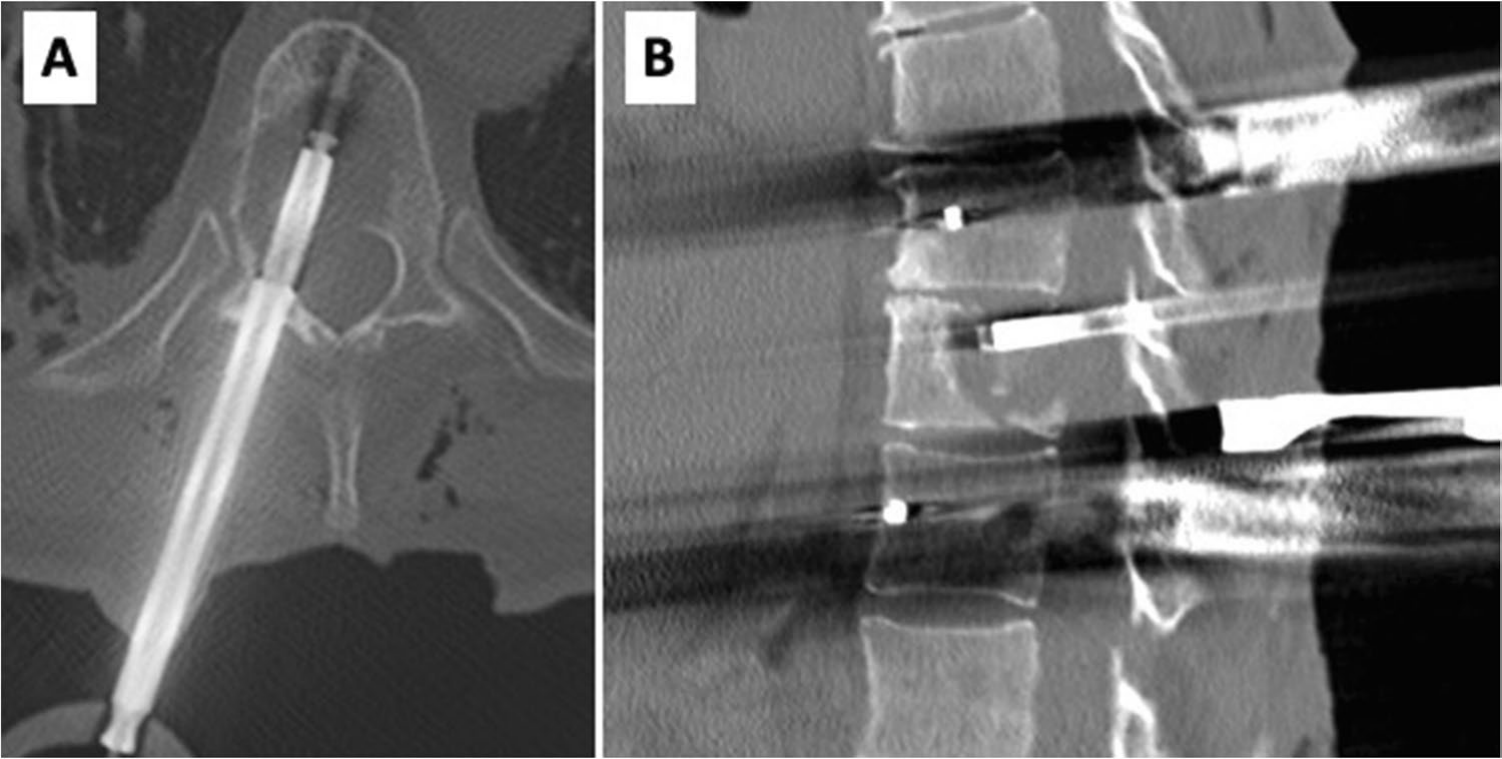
A) axial CT scan with intravertebral placement of guide and needle applicator in axial and B) sagittalplane
